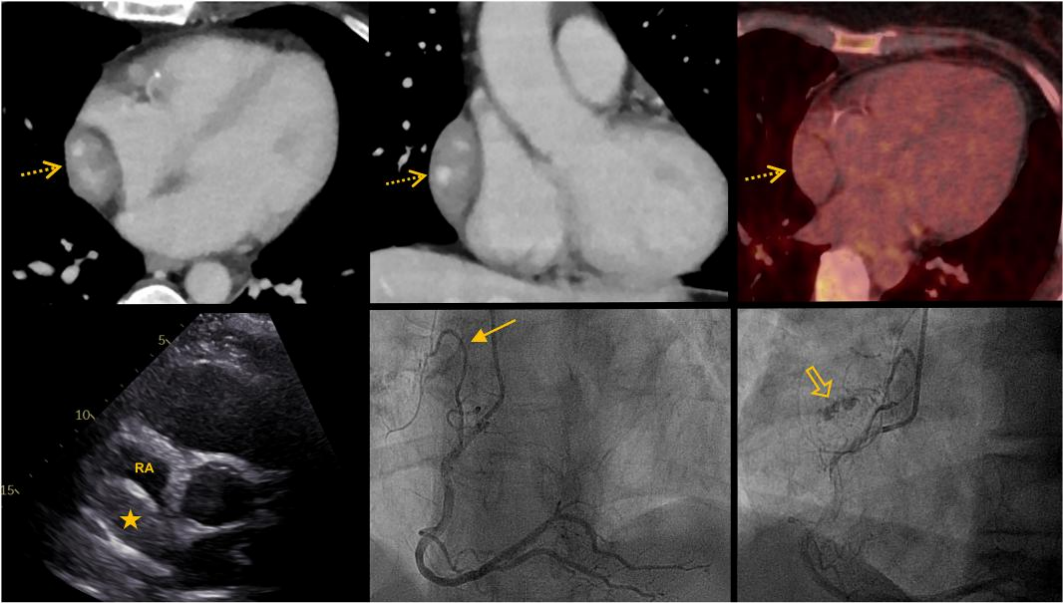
Multimodality imaging. Contrast-enhanced computed tomography in the portal venous phase in axial (*top* left) and coronal orientation (*top* middle) shows a well-demarcated, heterogeneously enhancing, ovoid mass adjacent to the right atrium (open arrows). Non-contrast positron emission tomography–computed tomography (*top* right) shows no significant fluorodeoxyglucose uptake in the pericardial mass (open arrow), instead remaining equivalent to the background blood pool activity. Transthoracic echocardiography in the parasternal short-axis view (*bottom* left) shows a well-demarcated, echogenic mass (star) adjacent to the roof of the right atrium. Coronary angiography showing blood supply to the tumour via an atrial branch of the right coronary artery (solid arrow; *bottom* middle) and ‘tumour blush’ of the mass (wide arrow; *bottom* right).
